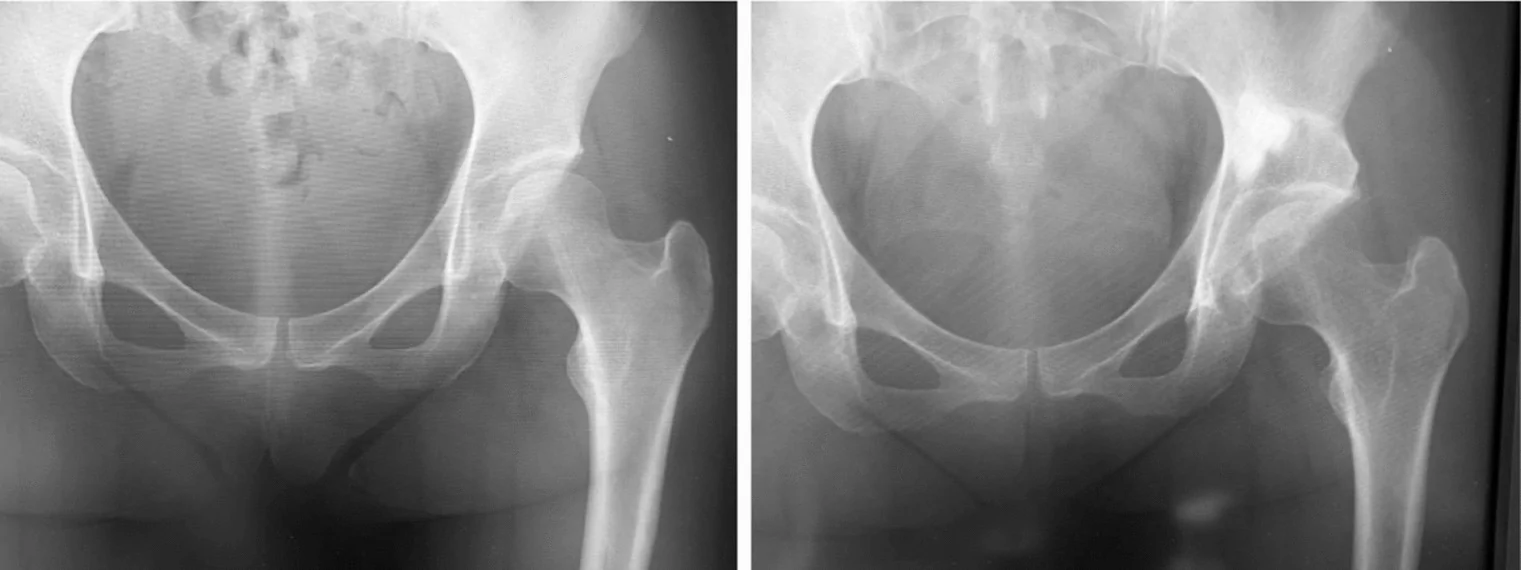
Preoperative and postoperative radiographs of a representative spherical periacetabular osteotomy (SPO) case. Unlike other procedures, osteotomies of the quadrilateral surface and pubis were not performed. The osteotomy gap was filled with a β-tricalcium phosphate bone substitute. Representative radiographs provided by Dr. Toru Nishiwaki with permission
